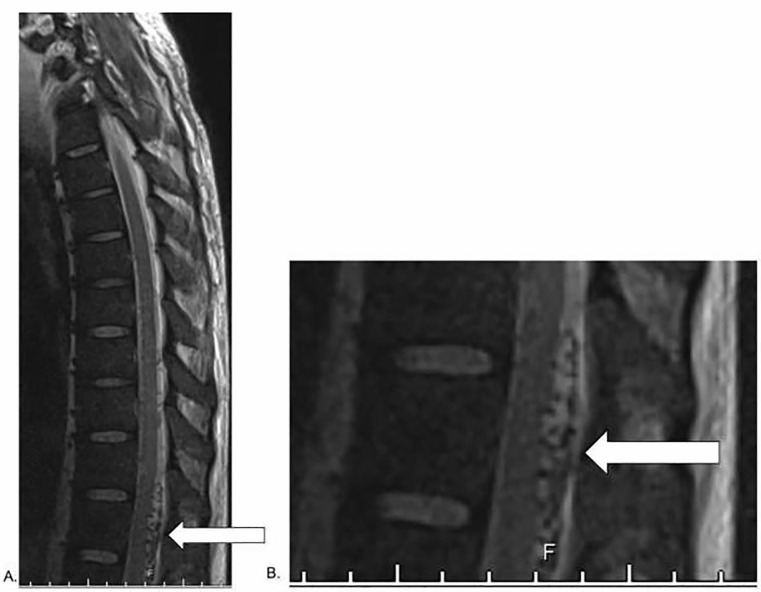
A) T2-weighted magnetic resonance imaging of the thoracic spine without
